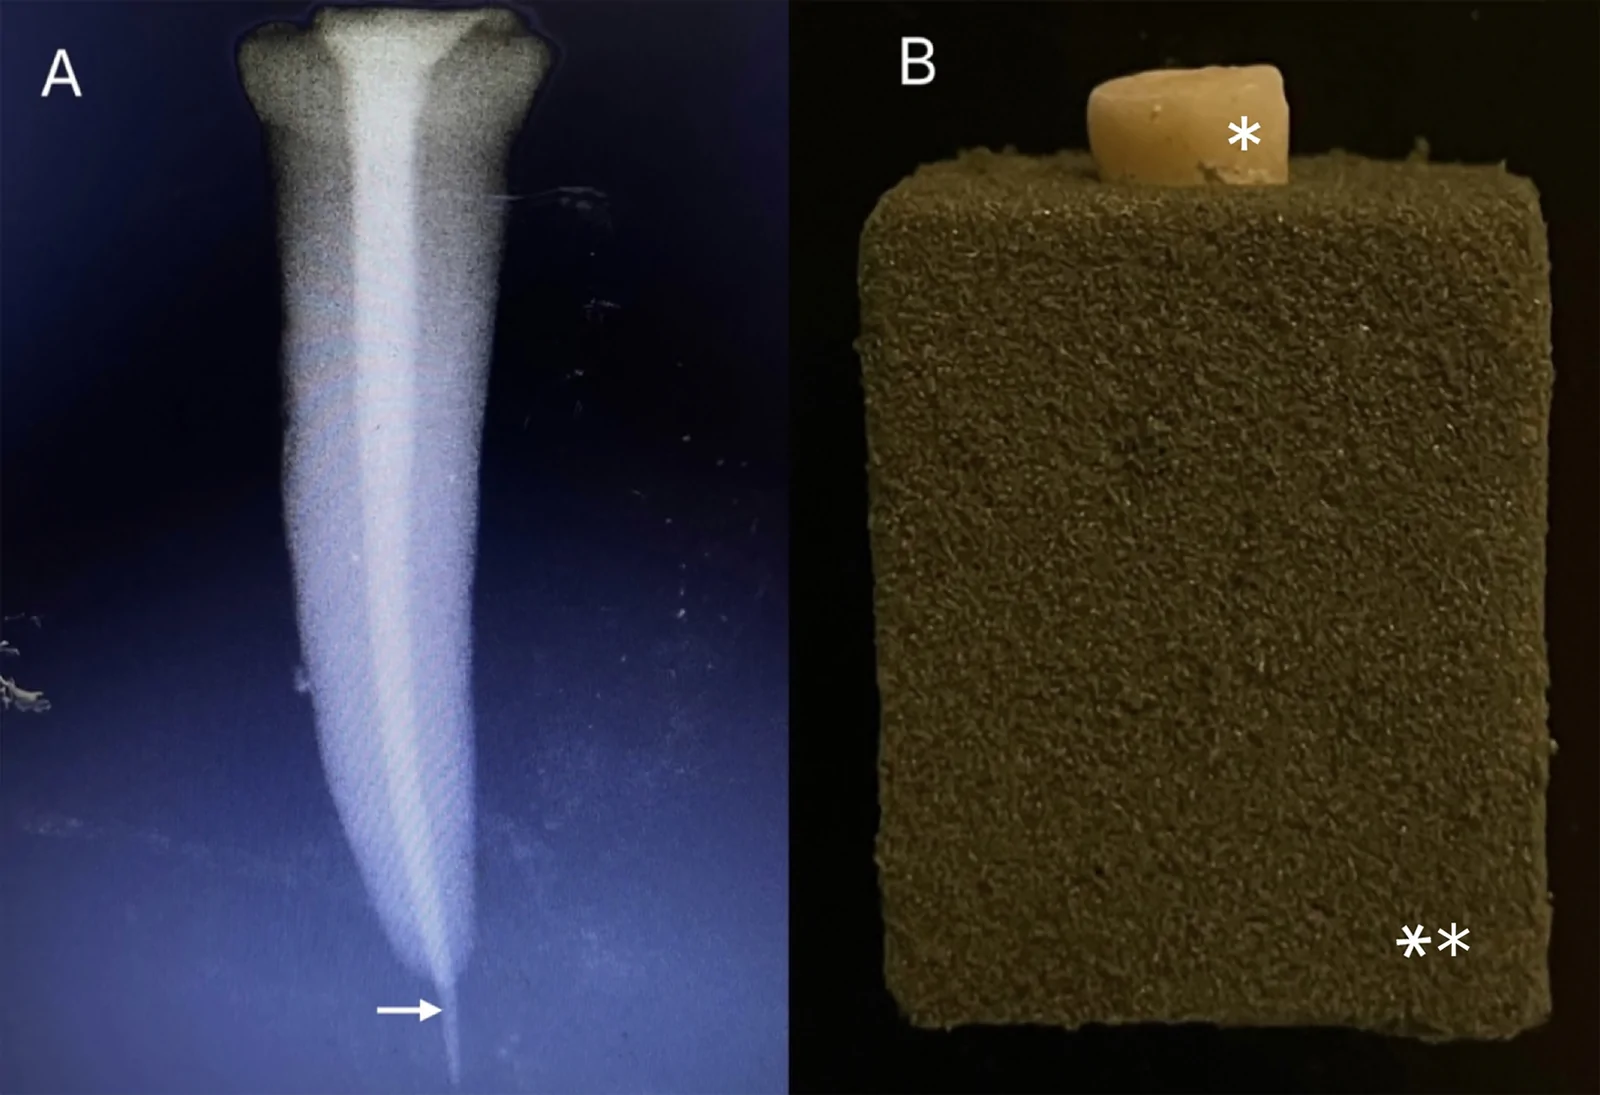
A Radiograph of the tooth after standardization and obturation with overextended filling, and (B) A tooth embedded in floral foam. (The arrow shows the 3 mm overextended gutta-percha) (* shows the tooth embedded in foam) ( shows the floral foam)
